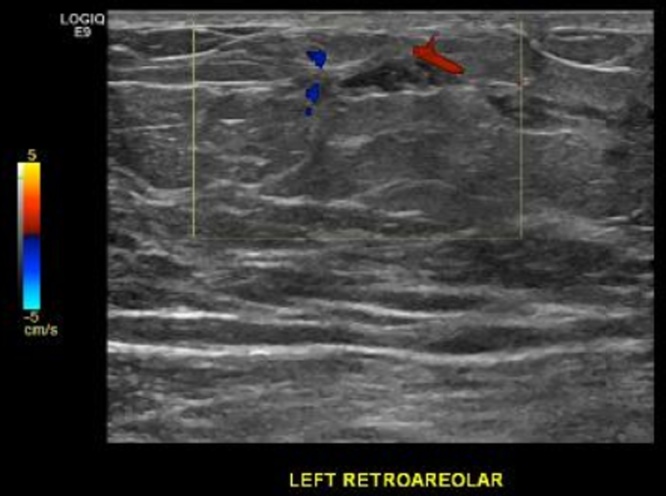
Ultrasound image of left retroareolar mass.
